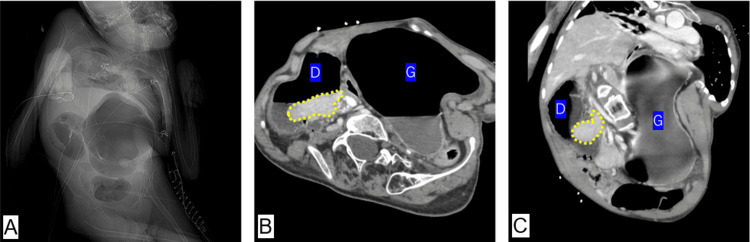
Contrast-enhanced abdominal CT ((A) scout, (B) axial, and (C) coronal views): severe scoliosis is noted, along with marked gastroduodenal distention and retrograde intussusception of the jejunum (outlined in yellow) into the horizontal segment of the duodenum. G: gastric; D: duodenum; CT: computed tomography
